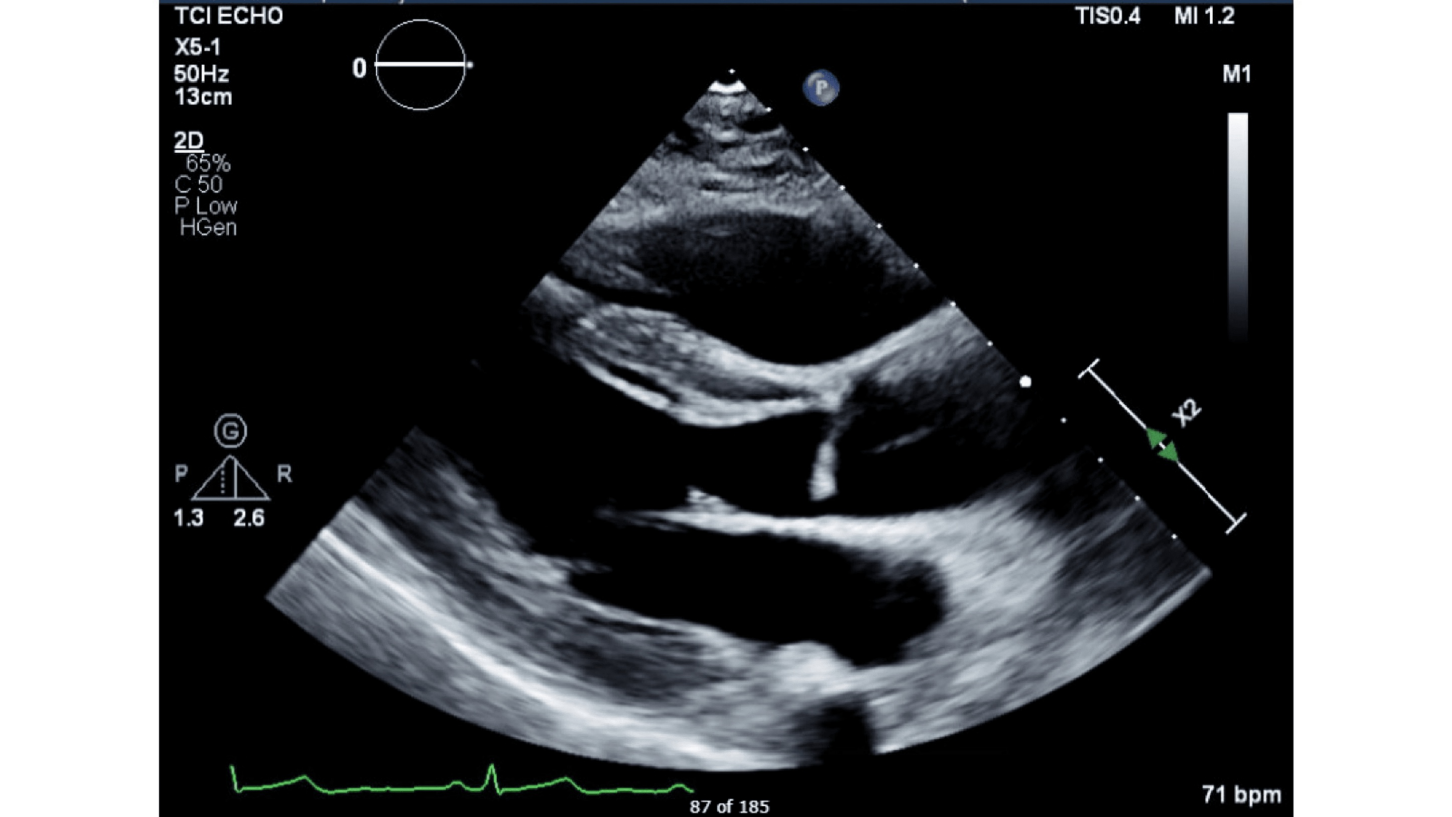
Follow-up transthoracic echocardiogram with parasternal long axis view with normal left ventricular wall motion and ejection fraction 55% to 60%.
